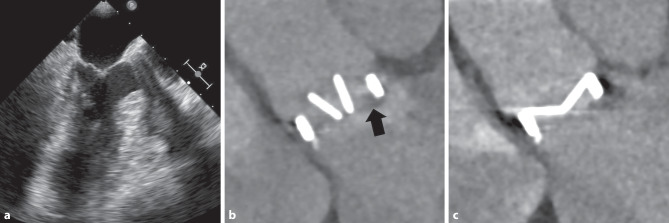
Thrombotic obstruction of a mechanical aortic valve diagnosed by CT. Images in a patient with a mechanical aortic valve (Carbomedics, size 25 mm), who presented with recurrent cortical strokes during episodes of subtherapeutic INR, caused by thromboembolic cerebrovascular occlusion. Transthoracic and transesophageal (panel a) echocardiography were inconclusive due to acoustic shadowing from the mechanical PHV, precluding definitive assessment of disc motion. Cardiac CT suggested prosthetic valve thrombosis, showing asymmetric disc opening (67.3 and 79.1°; panel b) that indicated impaired right disc mobility, symmetric disc closure, and a hypodense mass (arrowhead in panel b) adjacent to the sewing ring
